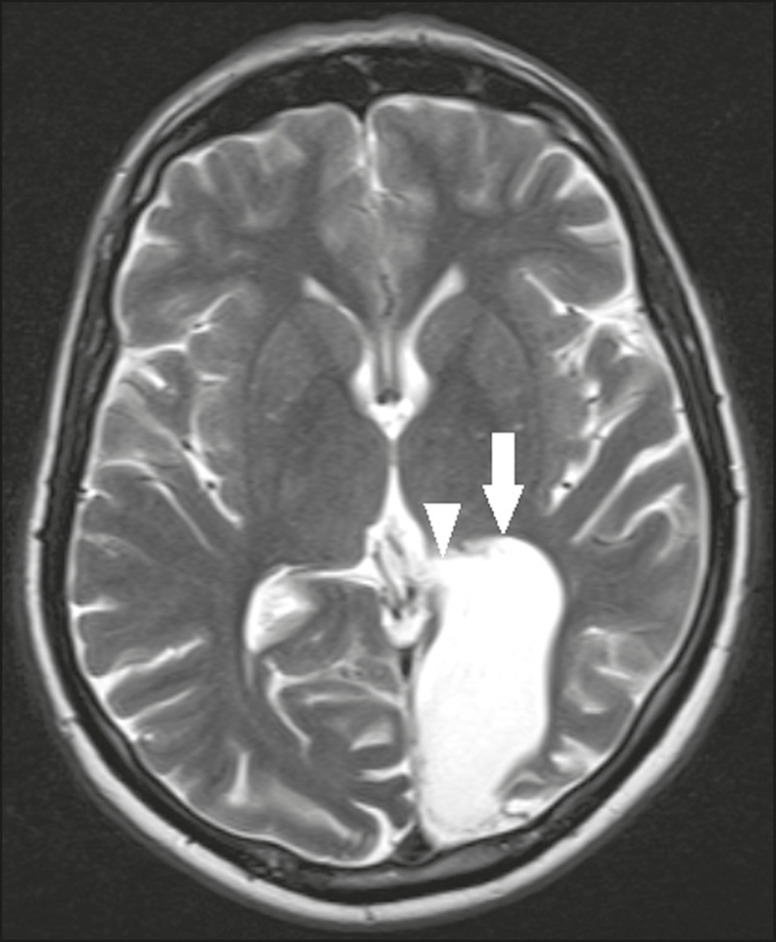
Porencephalic cyst. Axial T2-weighted MRI showing a cyst-like lesion in the left occipital lobe, with a signal intensity similar to that of the CSF (arrow). Note the communication with the ventricular system (arrowhead) and that the edges of the lesion are composed of white matter.
